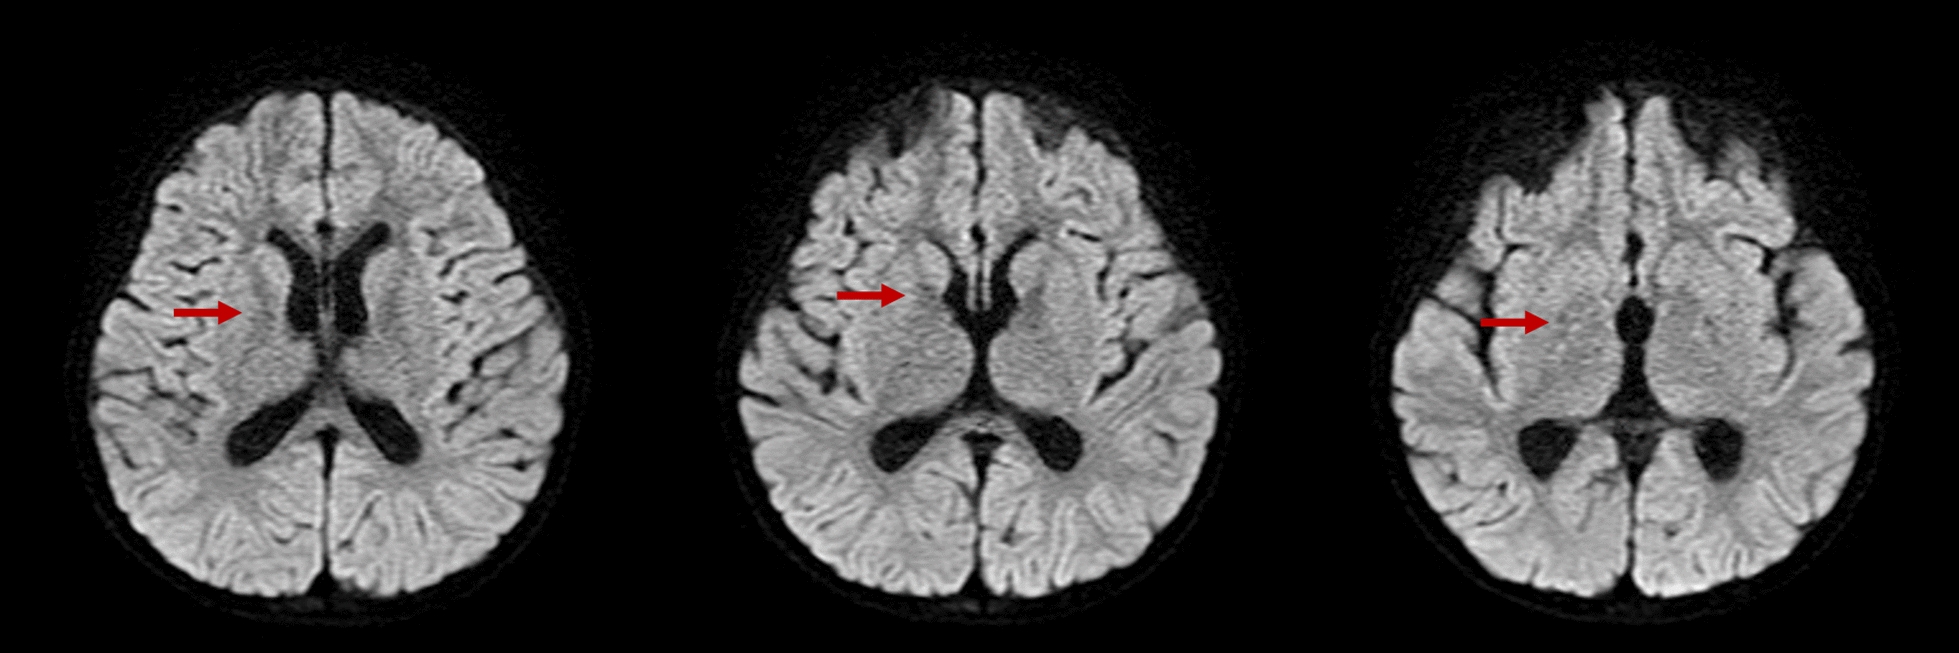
Brain MRI image of Case 9 at 7-years-old (DWI, axial view.). The arrows highlight the regions of encephalomalacia, which are prominently located in the bilateral lentiform nucleus, particularly affecting the globus pallidus
